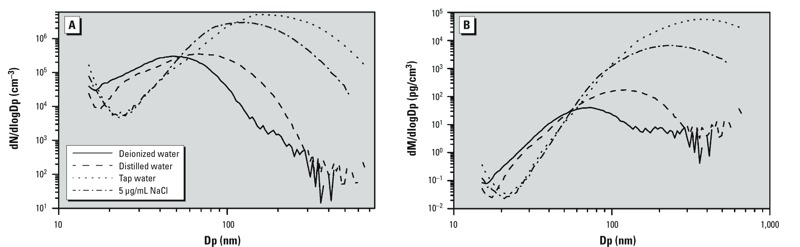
Number (*A*) and mass particle size (*B*) distributions determined for samples of deionized water, distilled water, tap water, and 5 μg/mL NaCl. Dp, particle diameter; NaCl, sodium chloride. Adapted from Knight and Petrucci (2003) and reprinted with permission from ACS Publications [*Analytical Chemistry.* Study of residual particle concentrations generated by the ultrasonic nebulization of deionized water stored in different container types, 75:4486–4492 (2003). Knight M, Petrucci GA. Copyright 2003 American Chemical Society].
